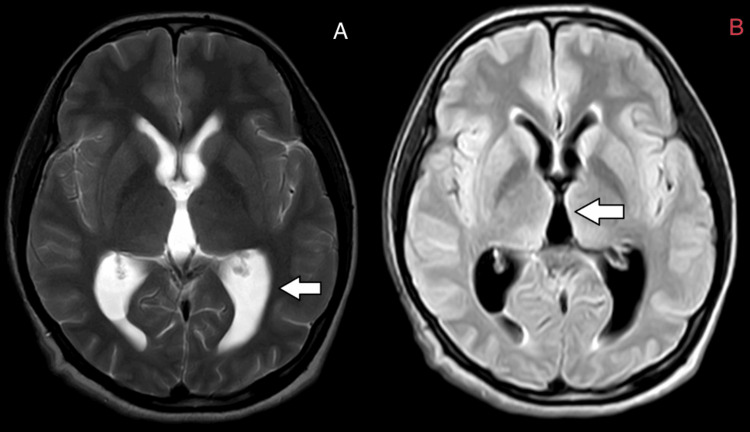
Axial T2 and FLAIR MRI brain images A) Arrow showing dilatation of the lateral ventricle; B) Arrow showing dilatation of the third ventricle. FLAIR: Fluid-attenuated inversion recovery; MRI: Magnetic resonance imaging
